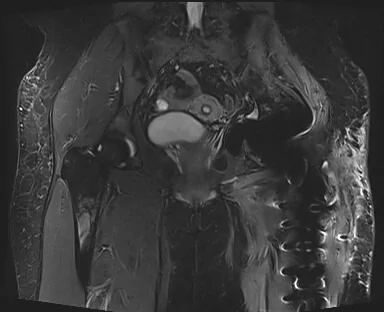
Conventional MRI sequences show obvious artifacts, making it impossible to evaluate the periprosthetic tissues.
